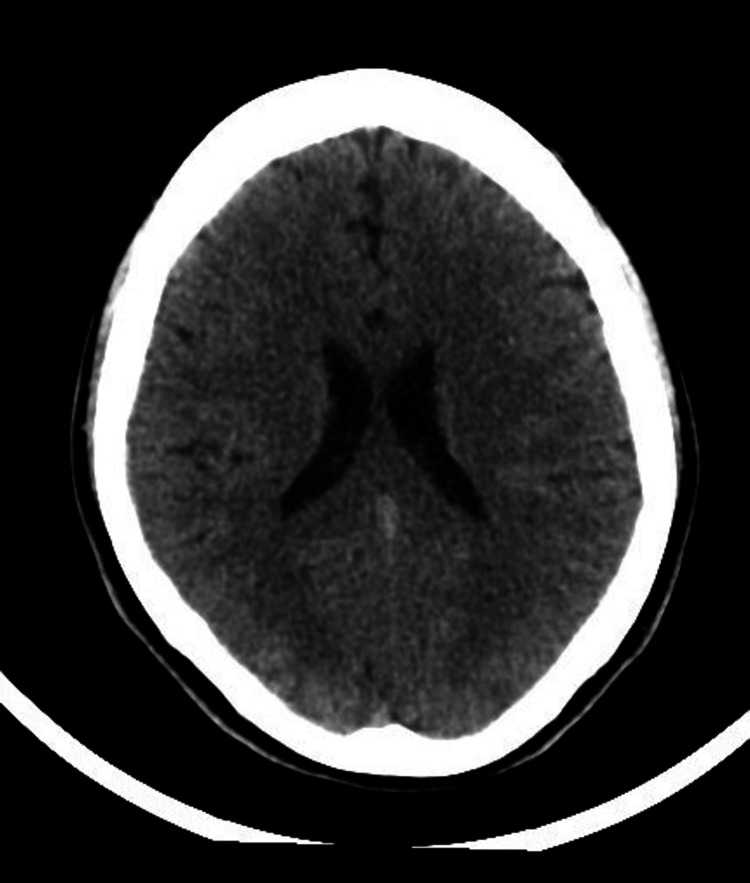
Brain CT scan view showed no evidence of intracranial hemorrhage, or focal mass lesions with the normal appearance of the ventricular system.
